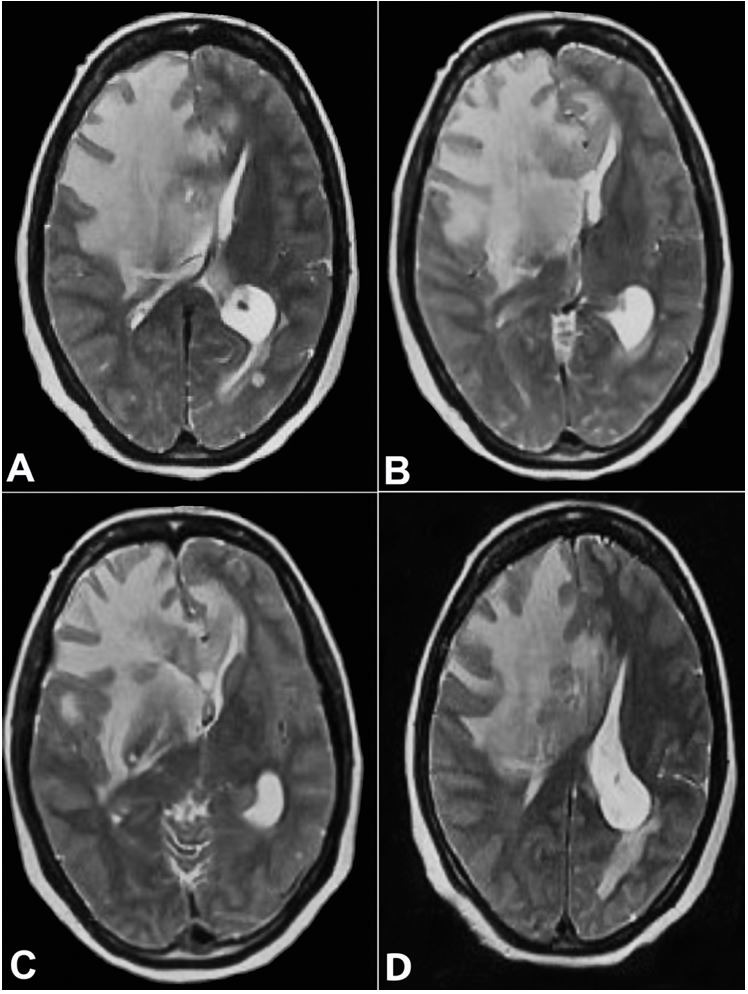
MRI axial T2 W image at the level of basal ganglia and body of lateral ventricle showing diffuse edema at the right frontal white matter and capsule-ganglionic region with expansion of frontal lobe, compression of ipsilateral lateral ventricle and contralateral midline shift along with focal areas intermediate signal intensity noted at the right peri-frontal region and corpus callosum.
